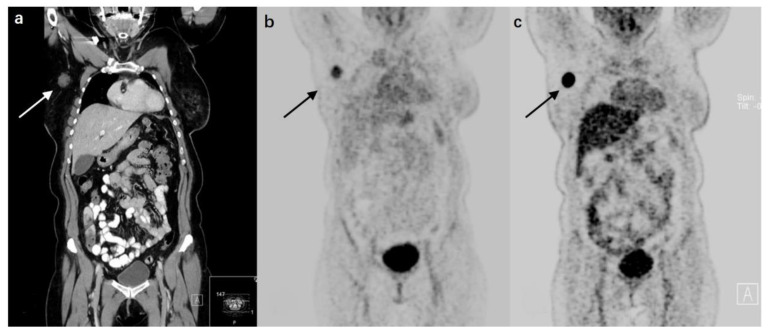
A 67-year-old patient with a nodal recurrence at 22 months after treatment from primary breast cancer. (a) Coronal CT reconstruction shows a contrast-enhancing lymph node metastasis with a diameter of 2.1 cm in the right axillary region. (b) The lesion is visually detectable on [^68^Ga]Pentixafor-PET (SUV_max_ = 4.0). (c) The lesion has a significantly higher [^18^F]FDG uptake (SUV_max_ = 24.4). (Reprinted from Ref. [27]).
